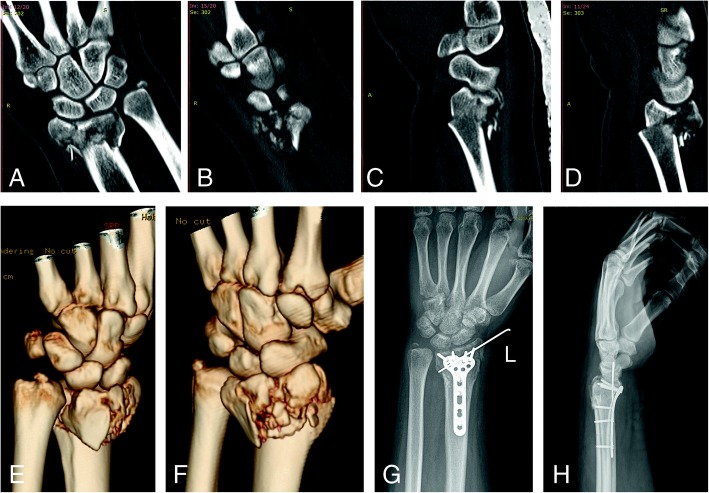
AO C3.1 fracture of the left distal radius occurred in a 37-year-old man due to motor vehicle collision injury. a–f Preoperative definite diagnosis of comminuted intra-articular fracture of the distal radius, by CT scanning and 3D reconstruction. g, h The satisfactory reduction and VLP fixation, in posteroanterior and lateral X-ray
